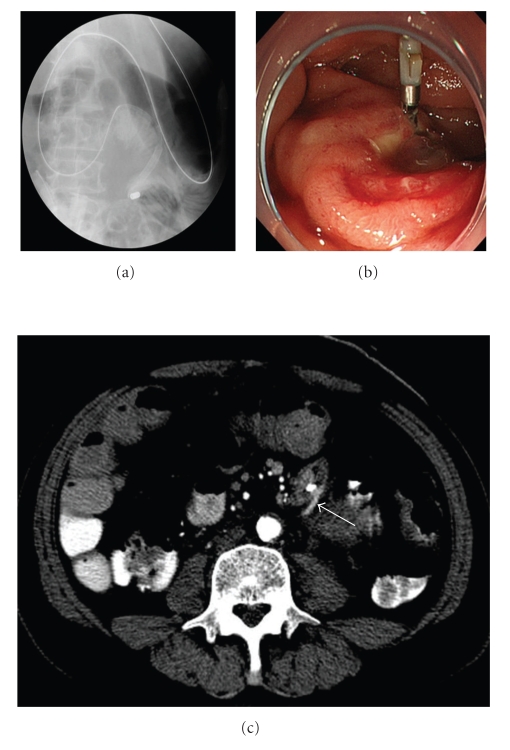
(a) A decompression tube was placed for postoperative ileus on day 3 post surgery. (b) Upper endoscopy shows an ulcerative lesion with pulsatile bleeding in the third portion of the duodenum. A marking clip was placed near the ulcer. (c) A computed tomography reveals that the marking clip and the stump of the SRA were very close (arrow).
